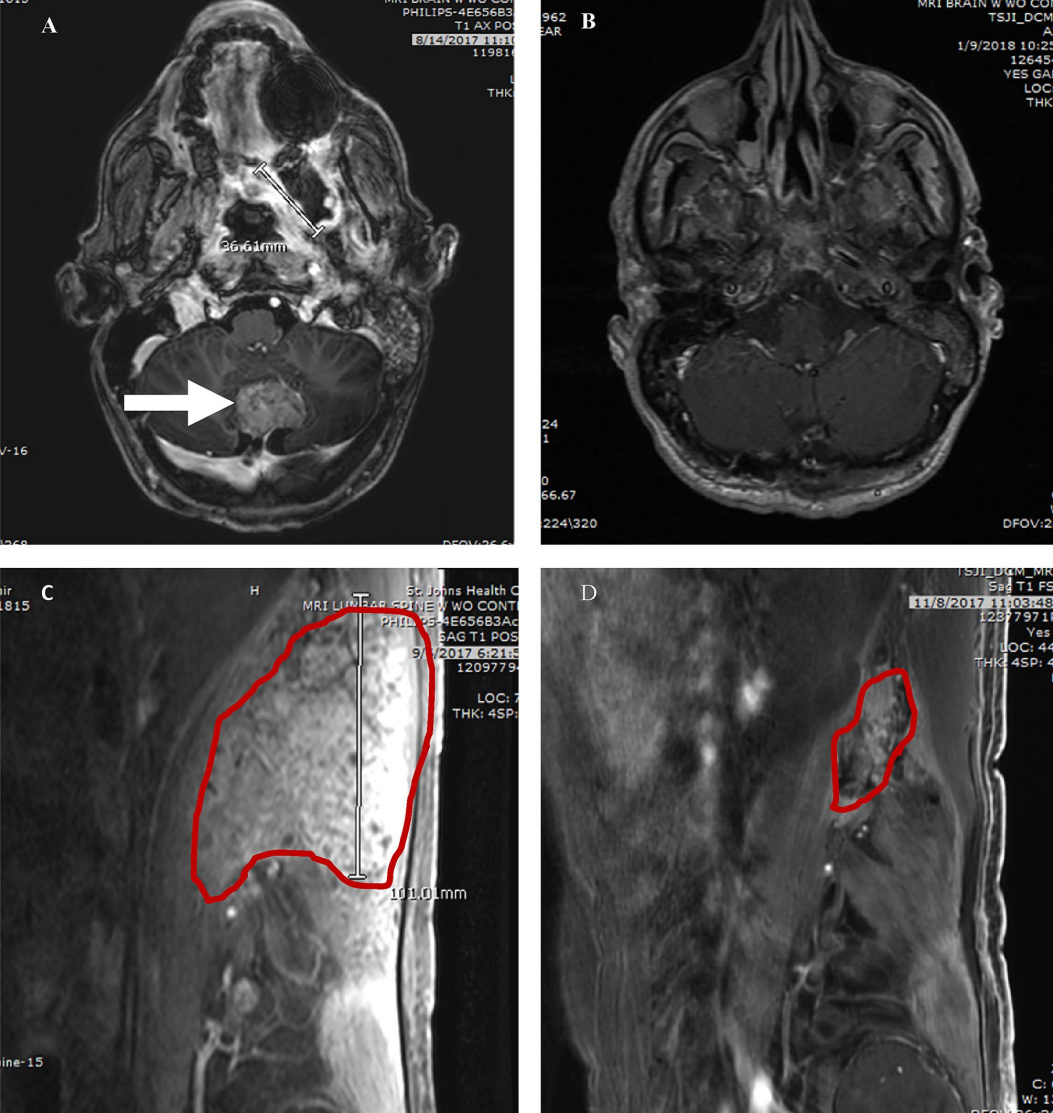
Case 1 MRI Brain and Retroperitoneum. Post-contrast MRI of patient with metastatic chordoma in brain (A) and retroperitoneum (C) before starting pemetrexed 900 mg/m^2^ and 5 months post-treatment in brain (B) and in 2 months retroperitoneum (D).
